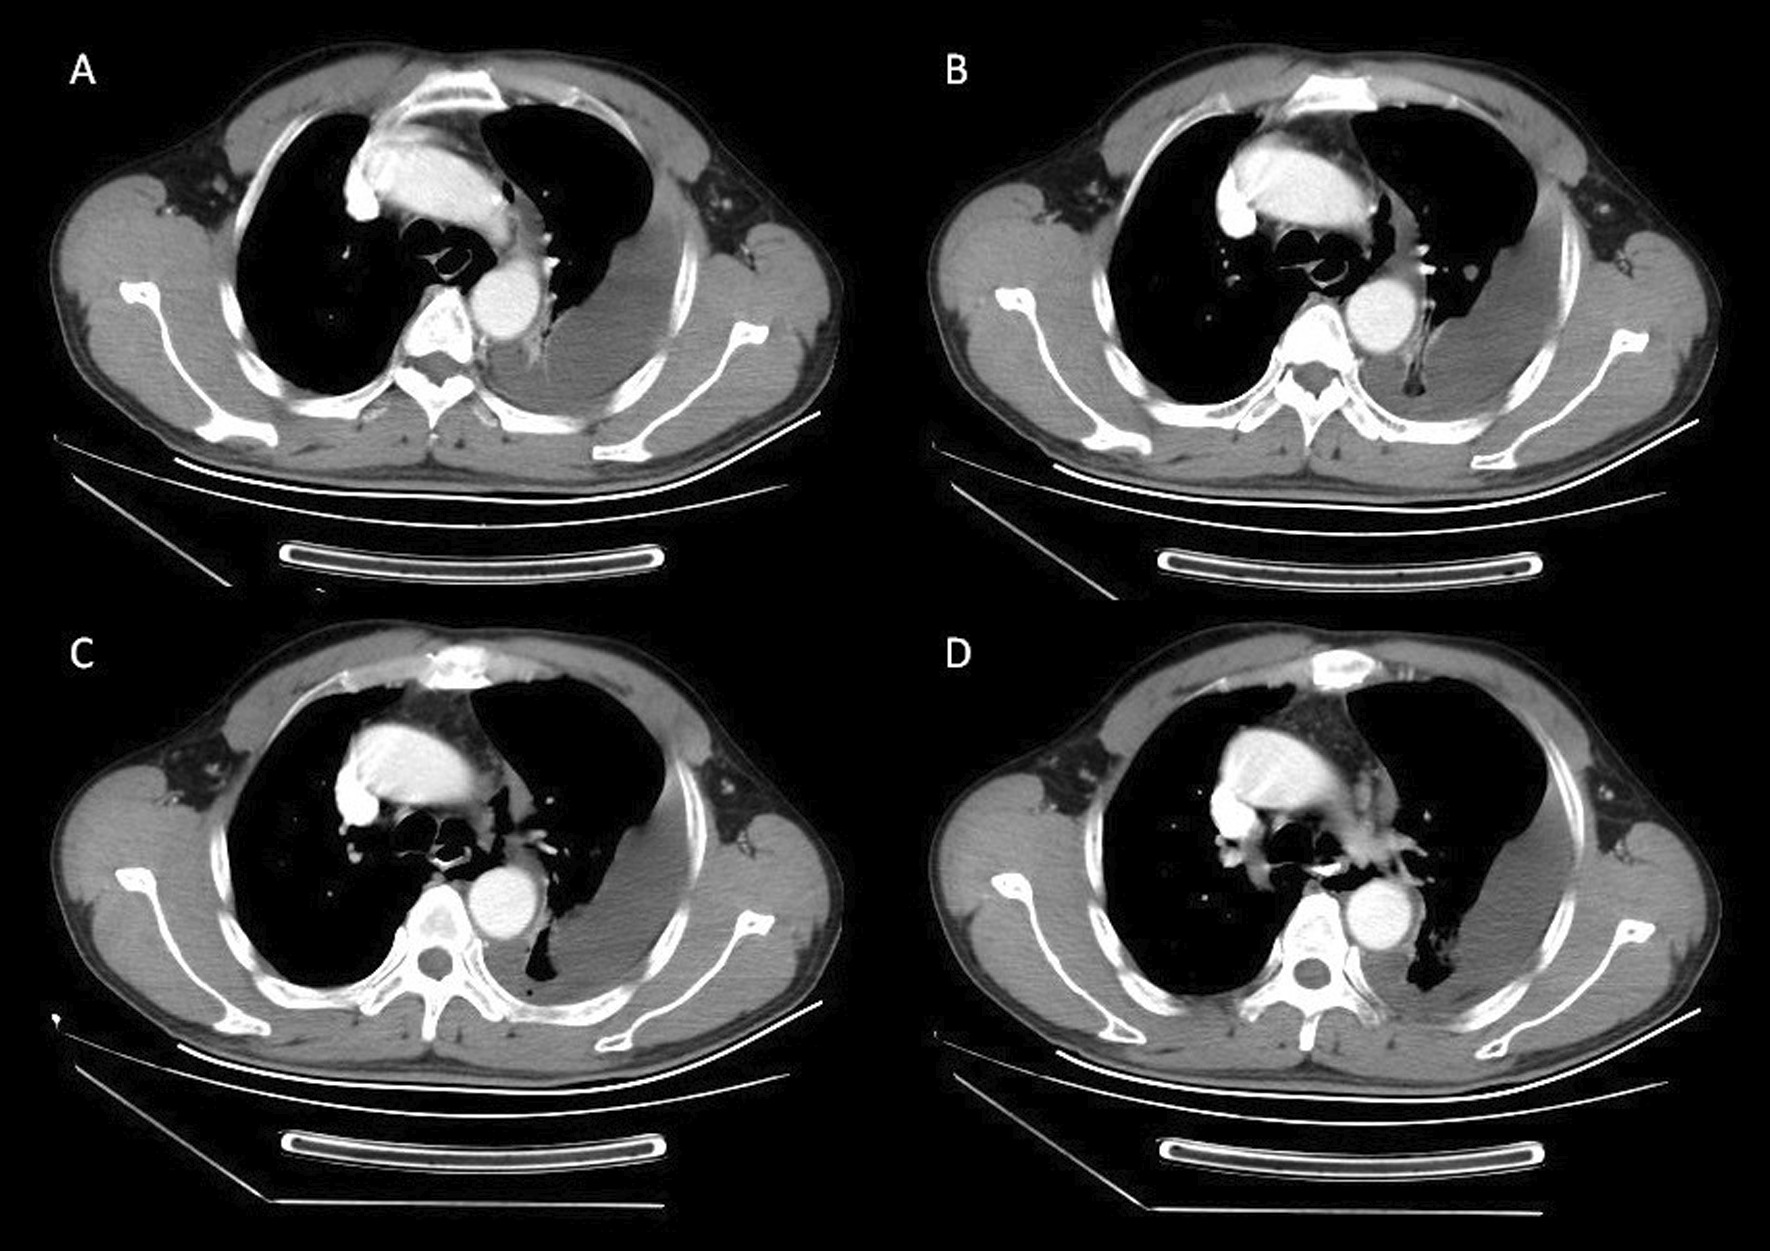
CT showing a tear in the left anterolateral wall of the distal esophagus, pneumomediastinum, and left pleural effusions
